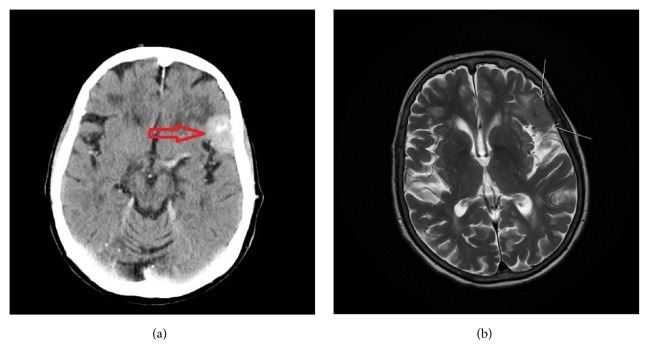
Imaging of meningioma of case II. (a) Contrast CT in the axial plane visualizing the meningioma (red arrow). (b) T2 weighted MRI in the axial plane visualizing the same meningioma (white arrows).
